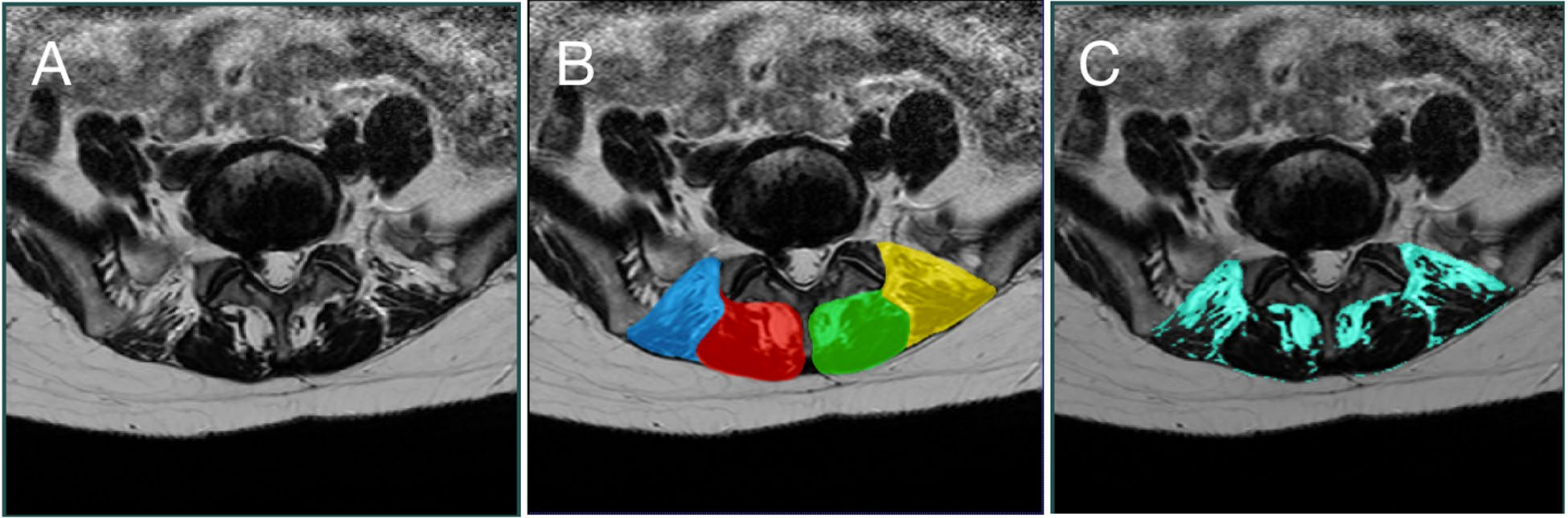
Demonstration of paraspinal muscle and fat tissue segmentation at the L5-S1 level. (A) Processed MRI, (B) manual segmentation of paraspinal muscles; (C) automatic fat tissue segmentation.
